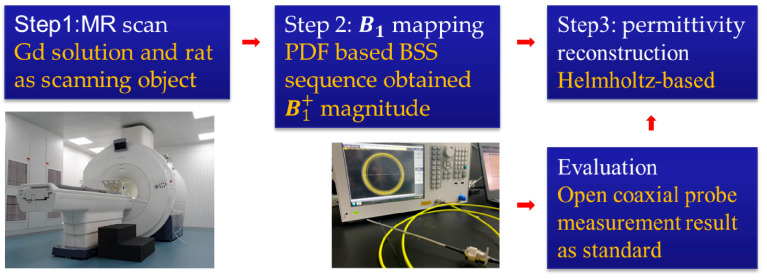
Scheme of the PDF-based channel-combination BSS for MR EPT, consisting of MR signal acquisition, the $B_{1}$ mapping technique, and permittivity reconstruction. Firstly, the MR image was obtained using the MR scanner. Then, the PDF-based channel-combination BSS sequence was performed to retrieve the $B_{1}^{+}$ magnitude needed for permittivity reconstruction. After that, the Helmholtz-based EPT reconstruction method was used to image the permittivity maps. Finally, the permittivity, measured by the open-ended coaxial probe method, was used as the ground-truth value to validate the accuracy of the EPT reconstructed results.
